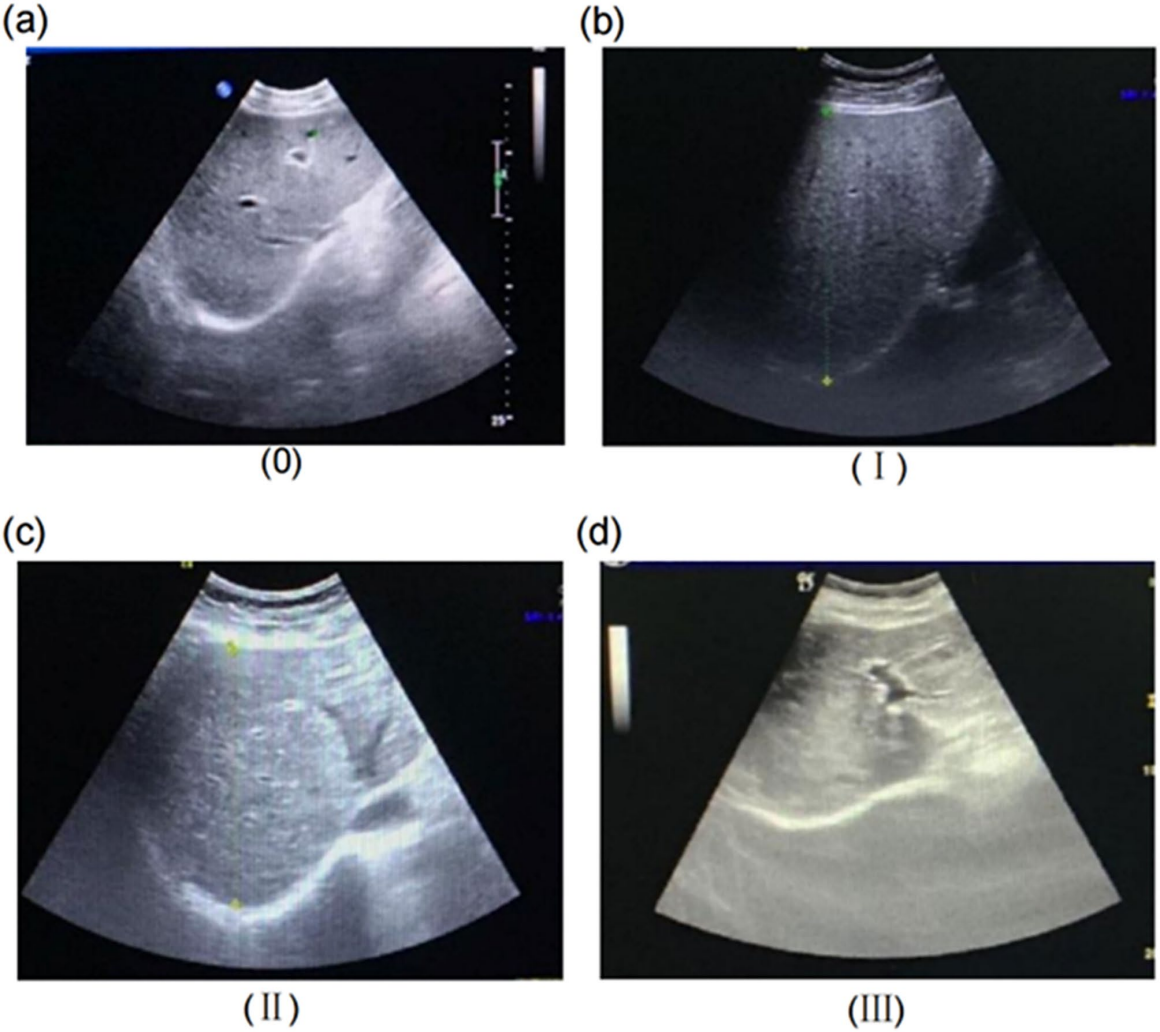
The hepatic fibrosis was graded from 0 to III: (a) Grade 0: normal and thicker light spot type, normal liver sonogram or only thick liver parenchyma echo; (b) Grade I: focal echoes in the liver parenchyma are scattered without clear boundary; (c) Grade II: fish-scale and cobweb type, a few focal echo density areas < 20 mm; (d) Grade III: echo density bands form a continuous network, multifocal echo areas > 20 mm, masses with central fibrosis.
